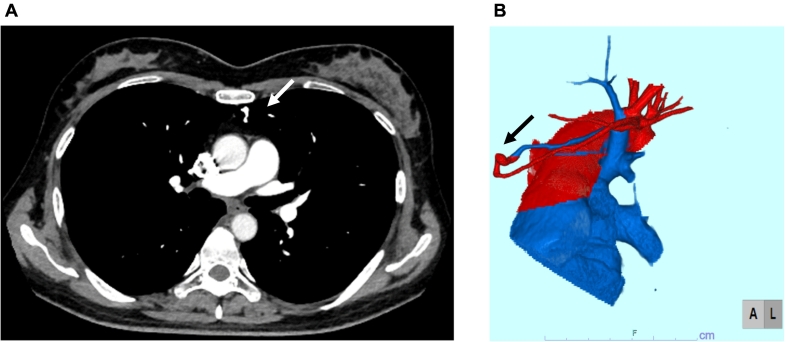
An abnormal vessel (2.2 mm in diameter) in the left pulmonary upper lobe on contrast computed tomography (arrows). (A) axial slice, (B) three-dimensional reconstruction of the pulmonary artery and vein.
